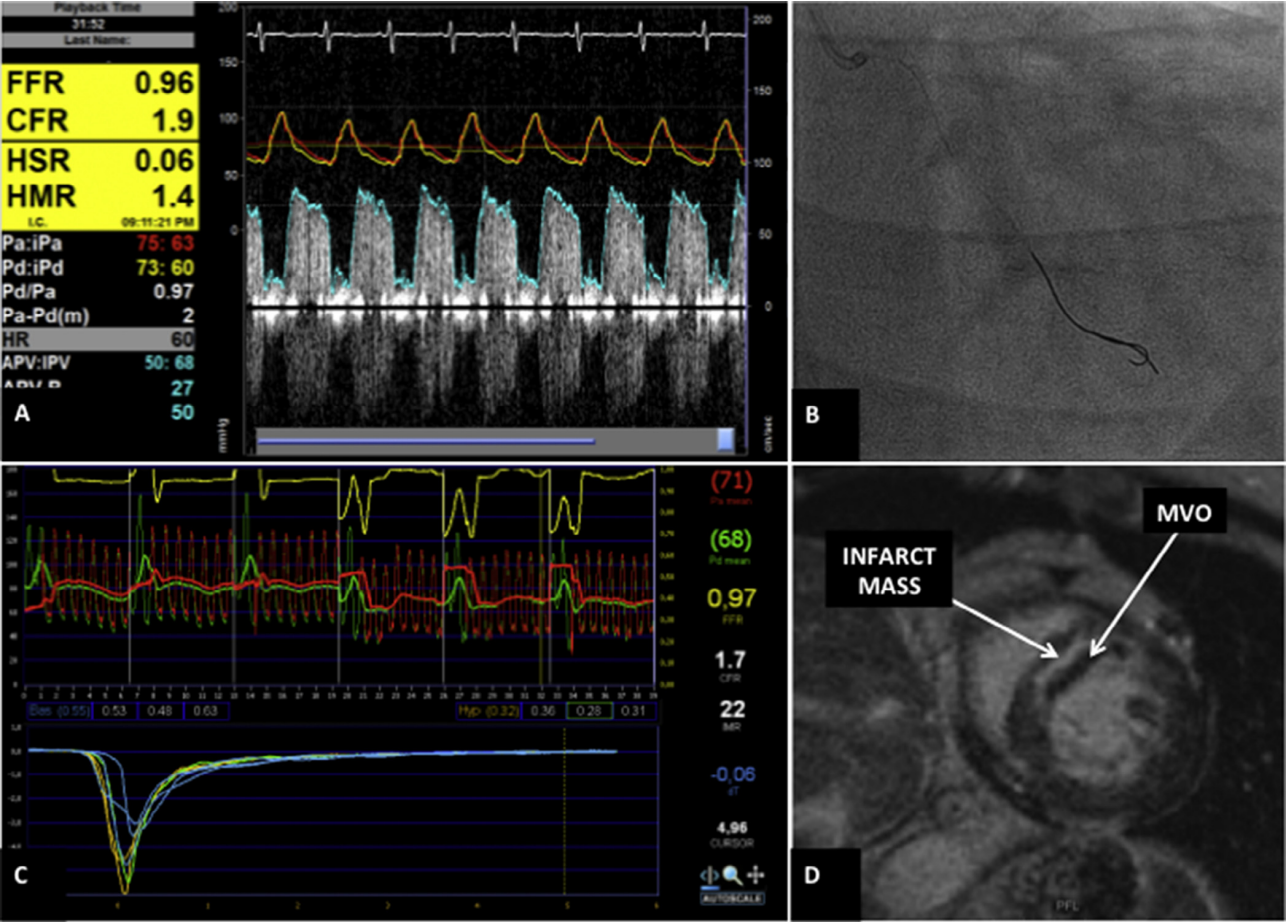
Cardiac catheterization protocol used to derive invasive measurements of microvascular resistance. *(A)* Combomap Console (Volcano Corporation, San Diego, California) displaying continuous aortic and P_d_ and Doppler flow velocity. *(B)* Coronary angiographic image demonstrating a 0.014-inch ComboWire (Volcano Corporation) and a 0.014-inch pressure wire (St Jude Medical, Uppsala, Sweden) placed in equivalent positions in the distal circumflex artery. *(C)* St Jude Console (St Jude Medical) displaying aortic and P_d_, and 3 T_mn_ measurements at both baseline and during steady-state hyperemia. *(D)* Late gadolinium enhancement cardiac magnetic resonance image 5 days after a revascularized acute ST-segment elevation myocardial infarction of the left anterior descending coronary artery. This short-axis view shows a hypoenhanced core of MVO within a hyperenhanced area of infarcted tissue in the anteroseptal myocardium. hMR = hyperemic microvascular resistance; hSR = hyperemic stenotic resistance; iPa = instantaneous aortic pressure; iPd = instantaneous distal coronary artery pressure.
